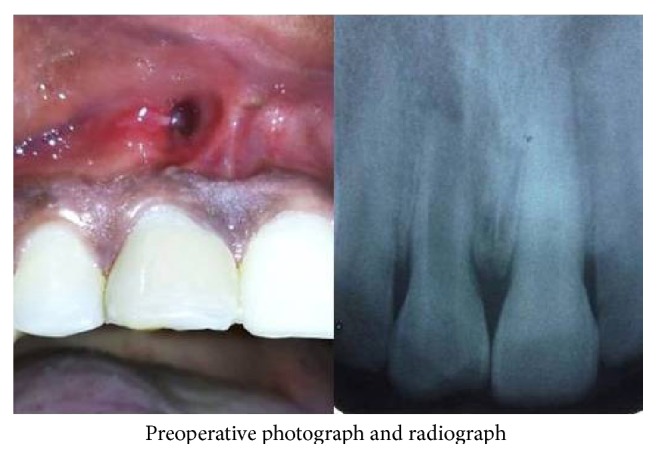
Preoperative photo- and radiograph.
